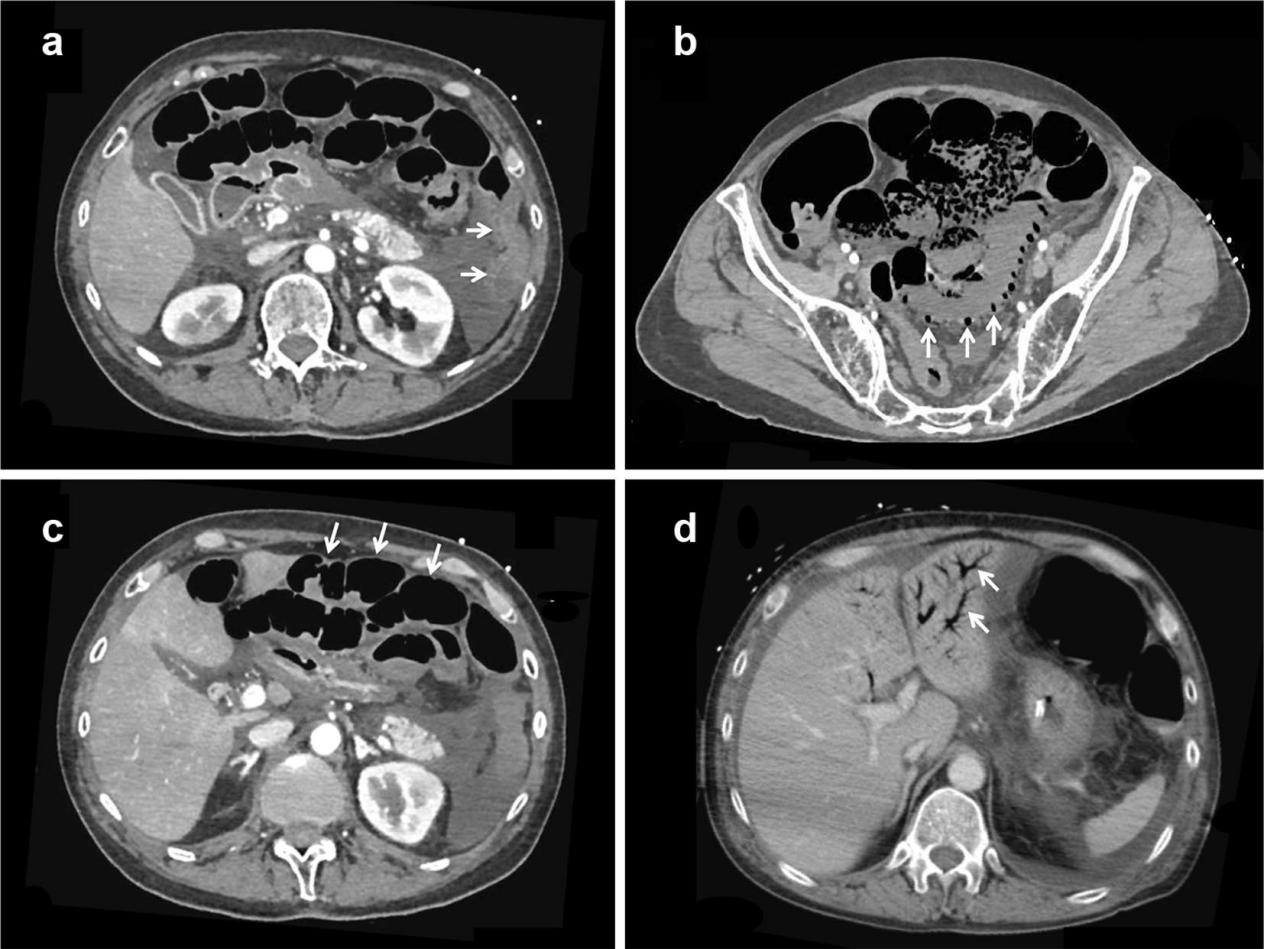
Representative CT-scan findings of non-occlusive mesenteric ischemia. a Absence of contrast-induced bowel wall enhancement (*arrows*). b Pneumatosis intestinalis and absence of contrast-induced bowel wall enhancement (*arrows*). c Bowel dilatation and absence of contrast-induced bowel wall enhancement (*arrows*). d Portal venous gas (*arrows*)
